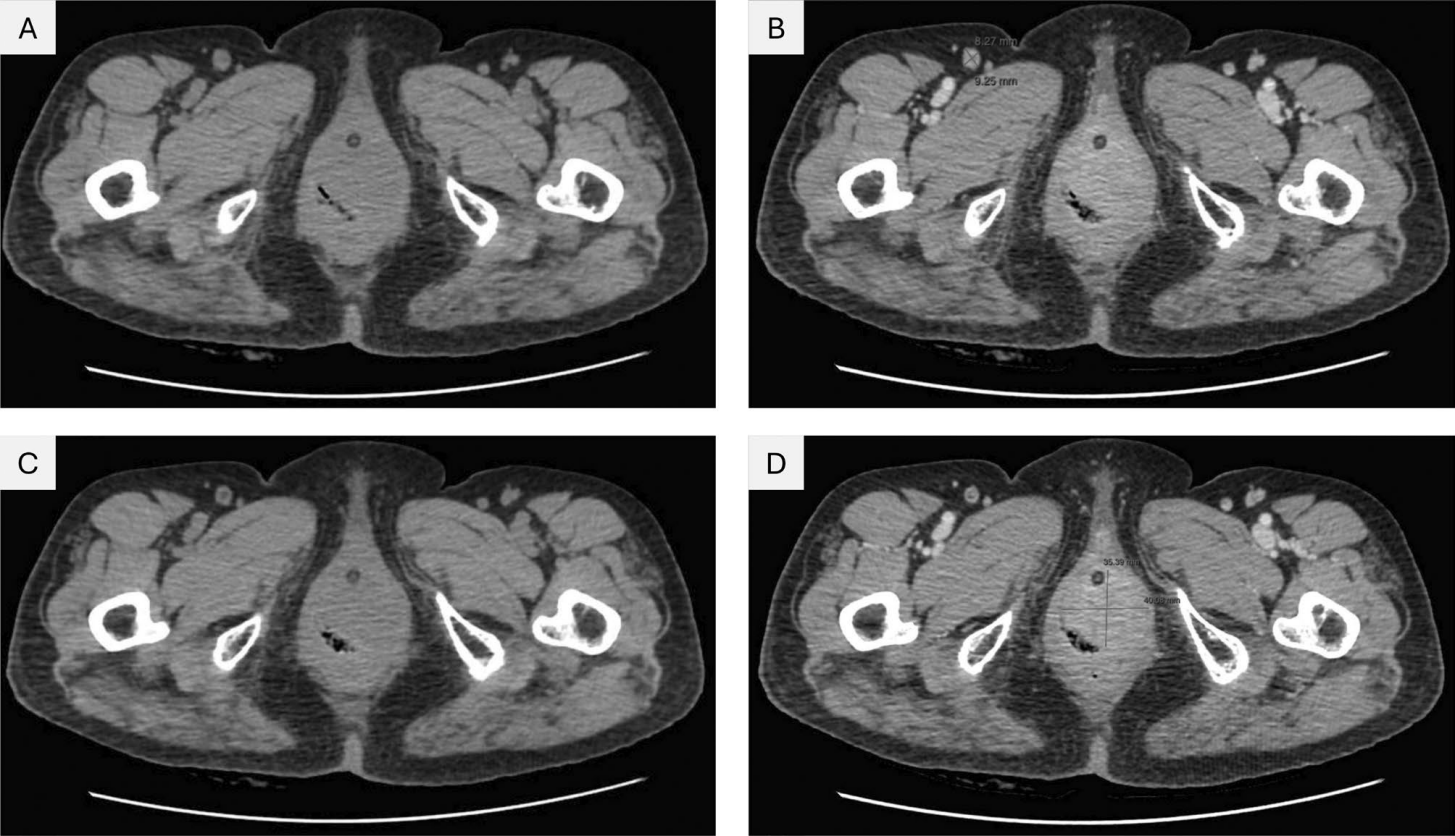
(A, B) Suspicious lymph node (LN) in the right groin on the pelvic CT scan (two crossed lines), before (left) and after (right) IV contrast injection. A well‐defined, rounded, 9.3‐mm LN with soft tissue attenuation is visible in the right groin, which is also heterogeneously and peripherally contrast‐enhanced. Overall, these features are indicative of malignancy, which was proven by an FNA‐based cytology report as LN‐metastasizing invasive carcinoma; (C, D) The labia minora solid lesion on the pelvic CT scan (two crossed lines), before (left) and after (right) IV contrast injection. This vulvar solid tumor (40 × 35 mm) is heterogeneously contrast‐enhanced and appears not to have invaded the urethra. The patient had a urethral catheter placed before undergoing CT scans to evaluate the extent of vulvar tumor invasion. CT, computed tomography; IV, intravenous; FNA, fine‐needle aspiration.
